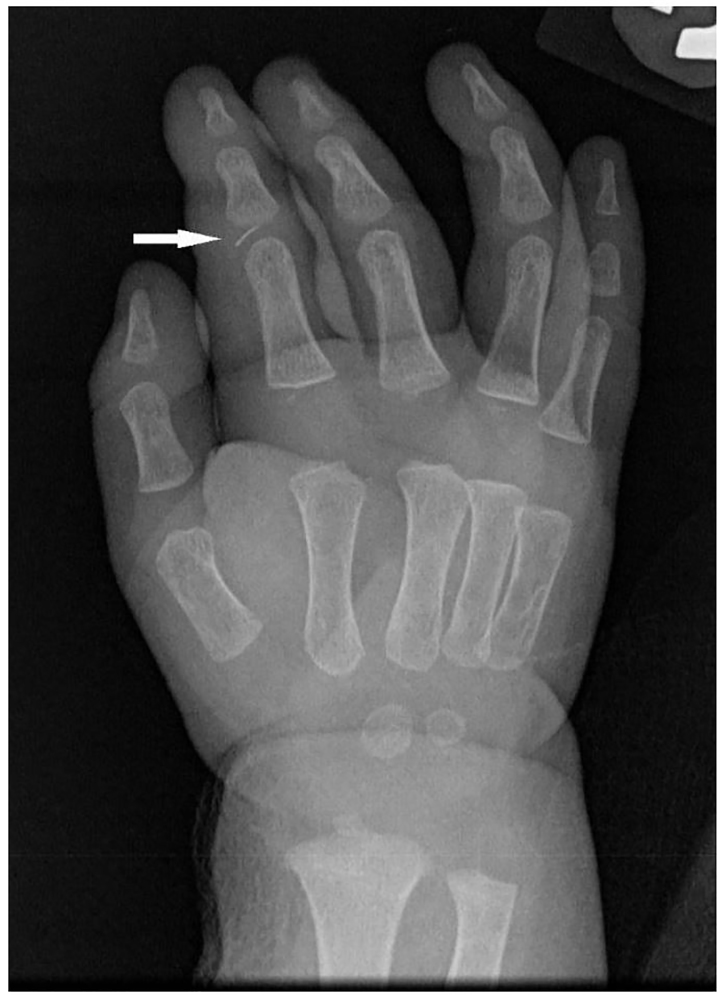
Radiograph of right hand: Note the foreign body within the index finger proximal inter-phalangeal joint (arrow).
